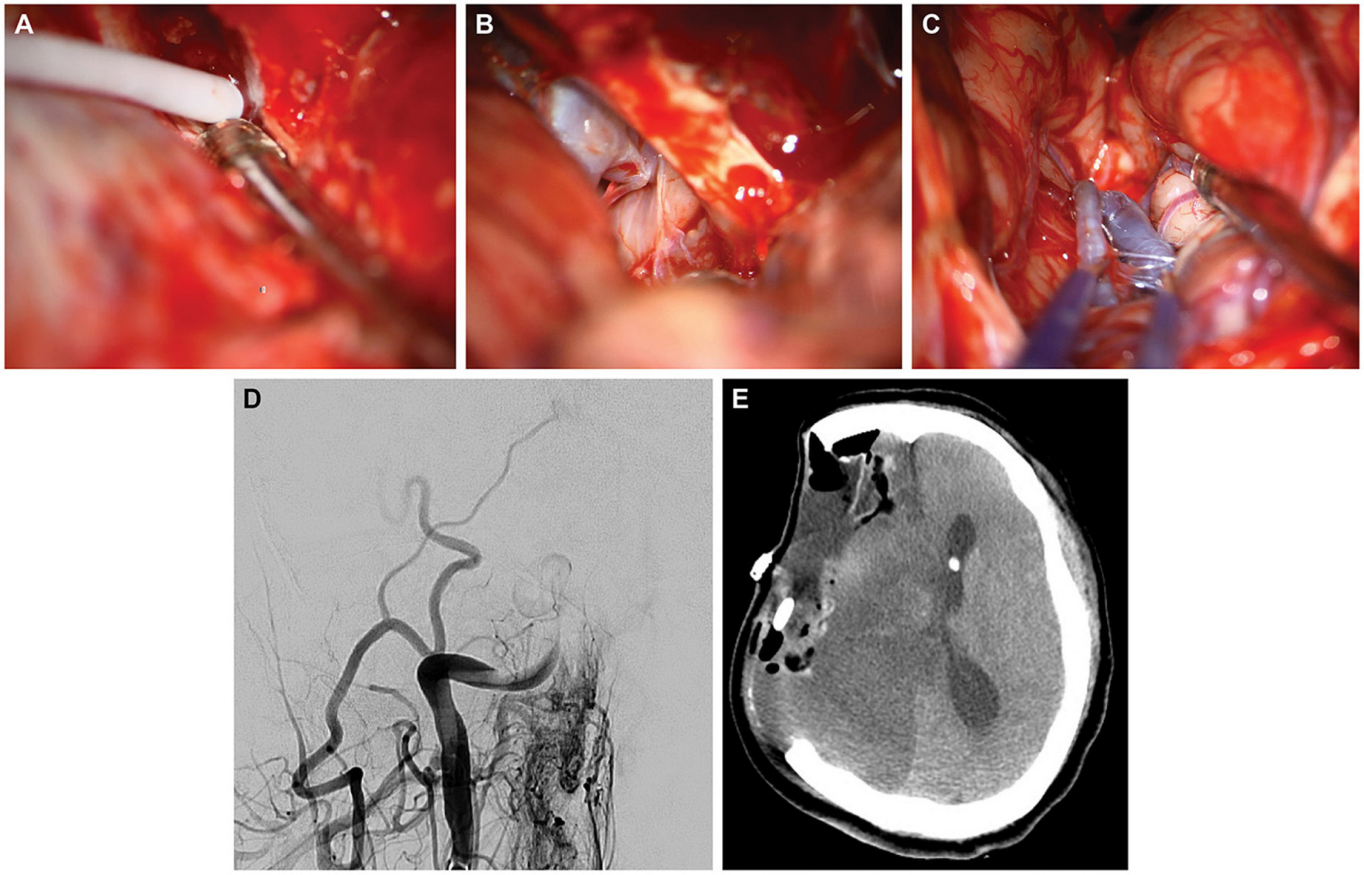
Case 2. Intraoperative photographs demonstrating (A) direct injection of fibrin glue into the cavernous sinus; (B) subsequent visualization of the patent internal carotid artery (ICA) during resection; and (C) 90 min later, the thrombosed middle cerebral artery, which has turned blue. (D) Postoperative posteroanterior angiography via the right common carotid artery demonstrating occlusion of the distal ICA. (E) Postoperative axial computed tomography of the head after decompressive hemicraniectomy and resection of ischemic territory. Used with permission from Barrow Neurological Institute, Phoenix, Arizona.
